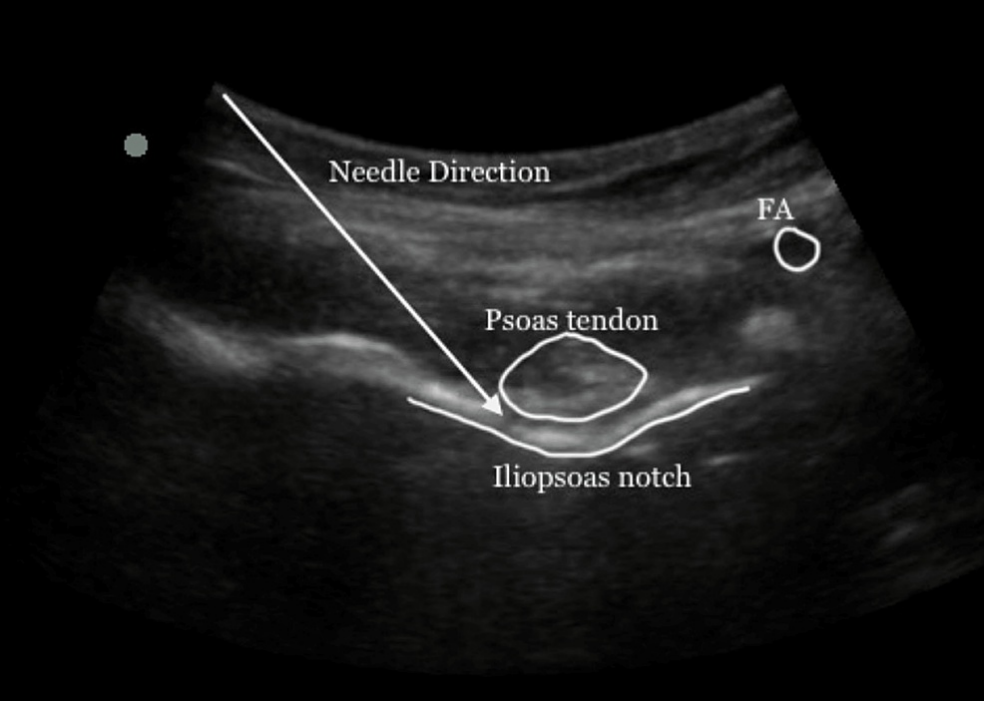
Ultrasound image of PENG block. The lateromedial approach of PENG block. The white arrow shows the direction of the needle in the plane between the psoas tendon and periosteum and between the anterior inferior iliac spine and iliopubic eminence or iliopsoas notch. FA: femoral artery, PENG: pericapsular nerve group
